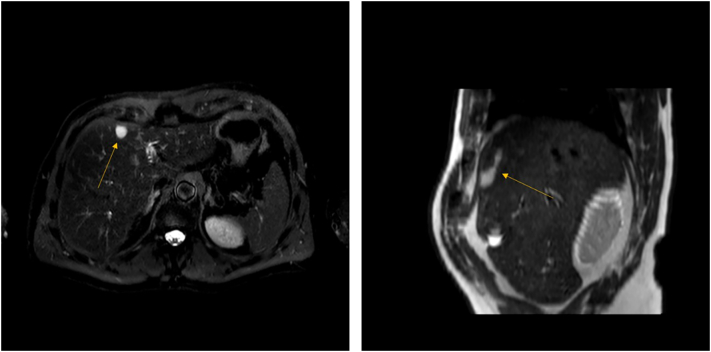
Transverse and sagittal abdominal magnetic resonance imaging of hepatic cyst located in segment IV of the liver (arrow).
